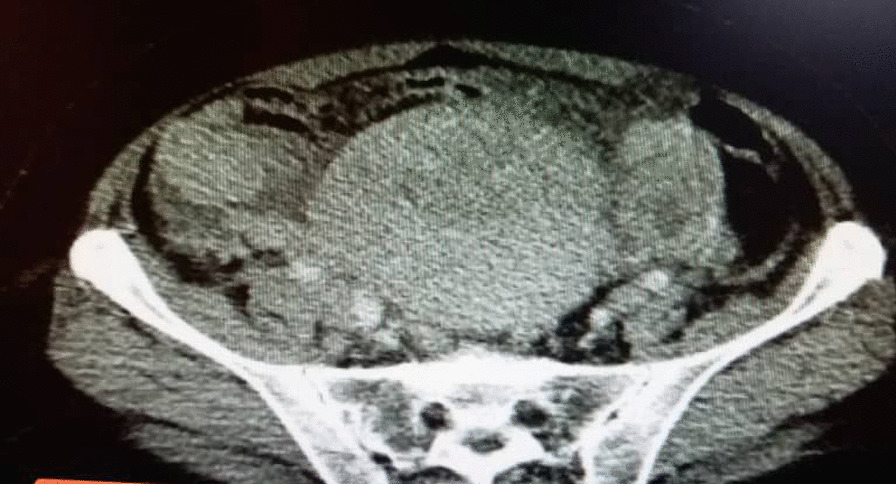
The computed tomography of the chest, abdomen, and pelvis after administering intravenous contrast agents showed a uterine sizable lobulated mass with irregular borders and high heterogeneous density, measuring about 112 mm × 191 mm × 160 mm, extending to the right and left ovaries
